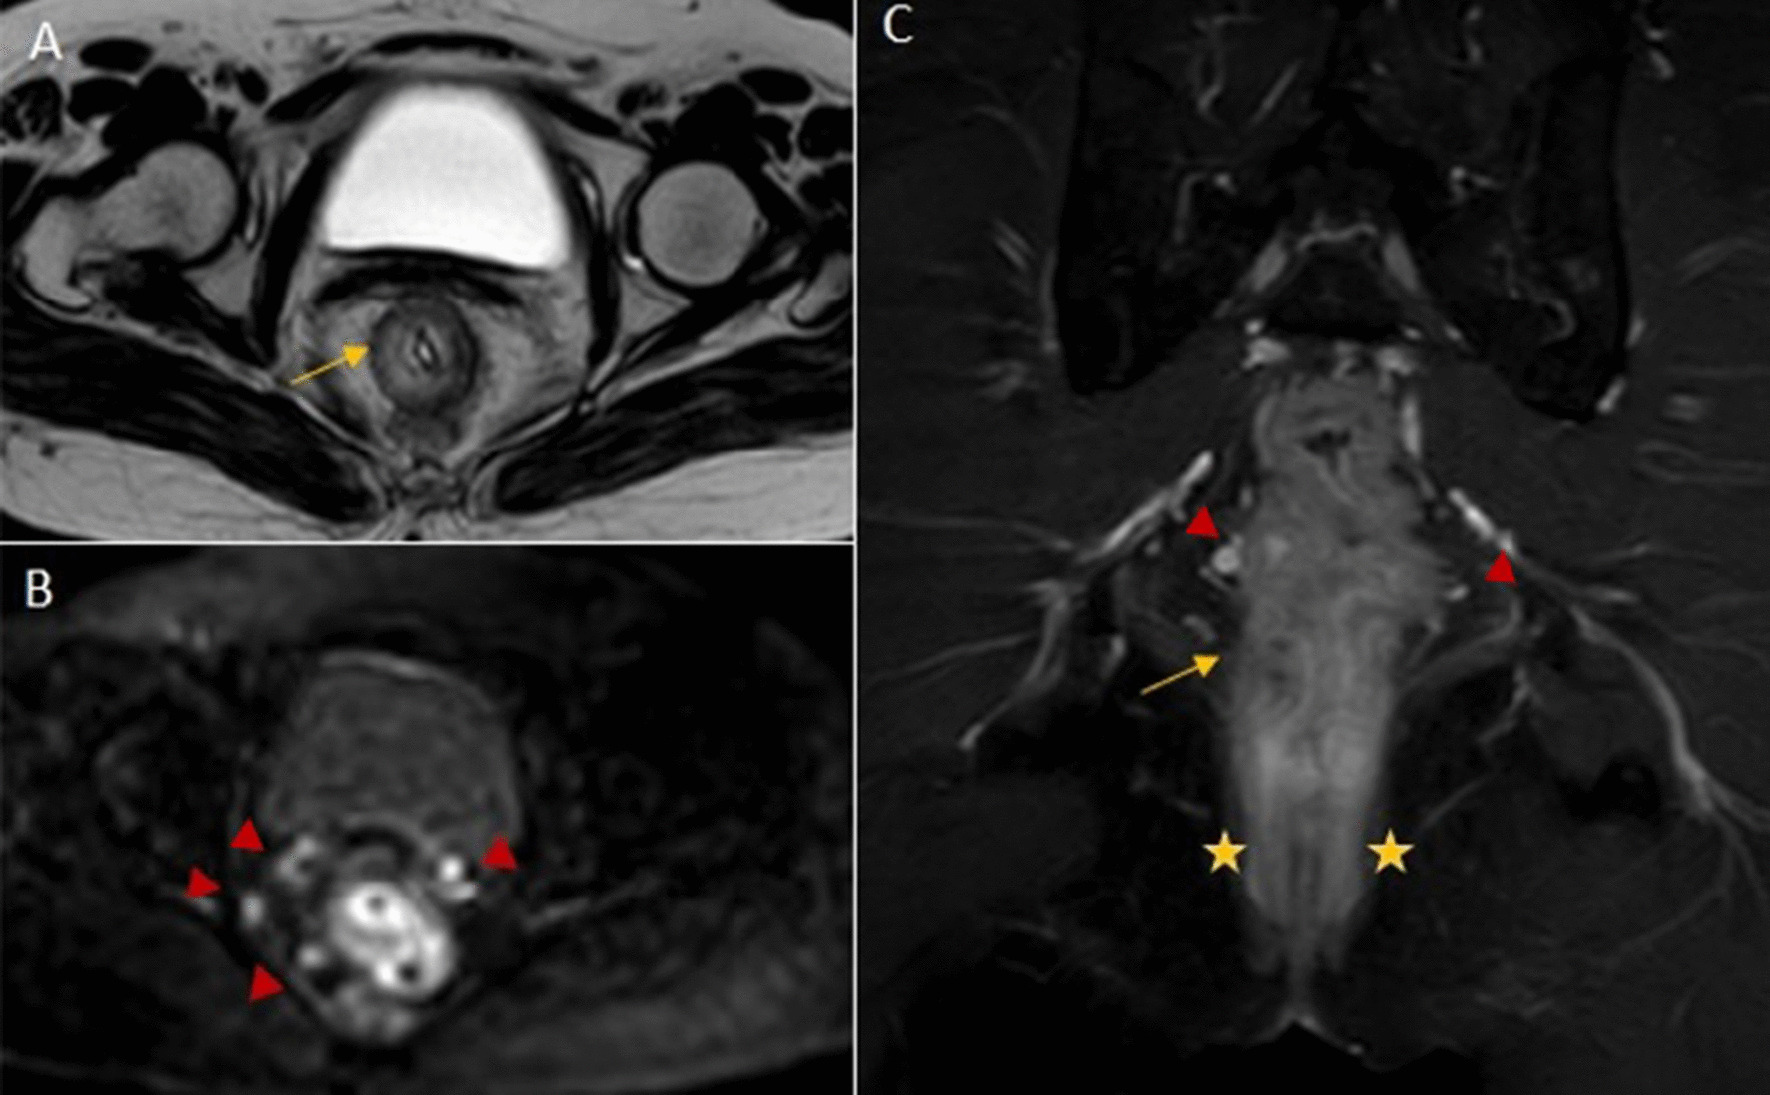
Pre and post contrast pelvic MRI: 36-year-old female post neoadjuvant rectal adenocarcinoma: Axial T2 weighted image (A), Axial diffusion weighted image (B) and coronal post contrast T1 weighted image with fat suppression (C): Circumferential irregular thickening of the lower third rectum (arrows) involving the external muscle layer with minimal extramural spread, no MRF involvement, extending downward into the internal anal sphincter sparing the external sphincter (asterisks). Multiple mesorectal rounded LNs (arrowheads) better detected on DW image, no extra-mesorectal LNs. MRI based staging T3, N2
